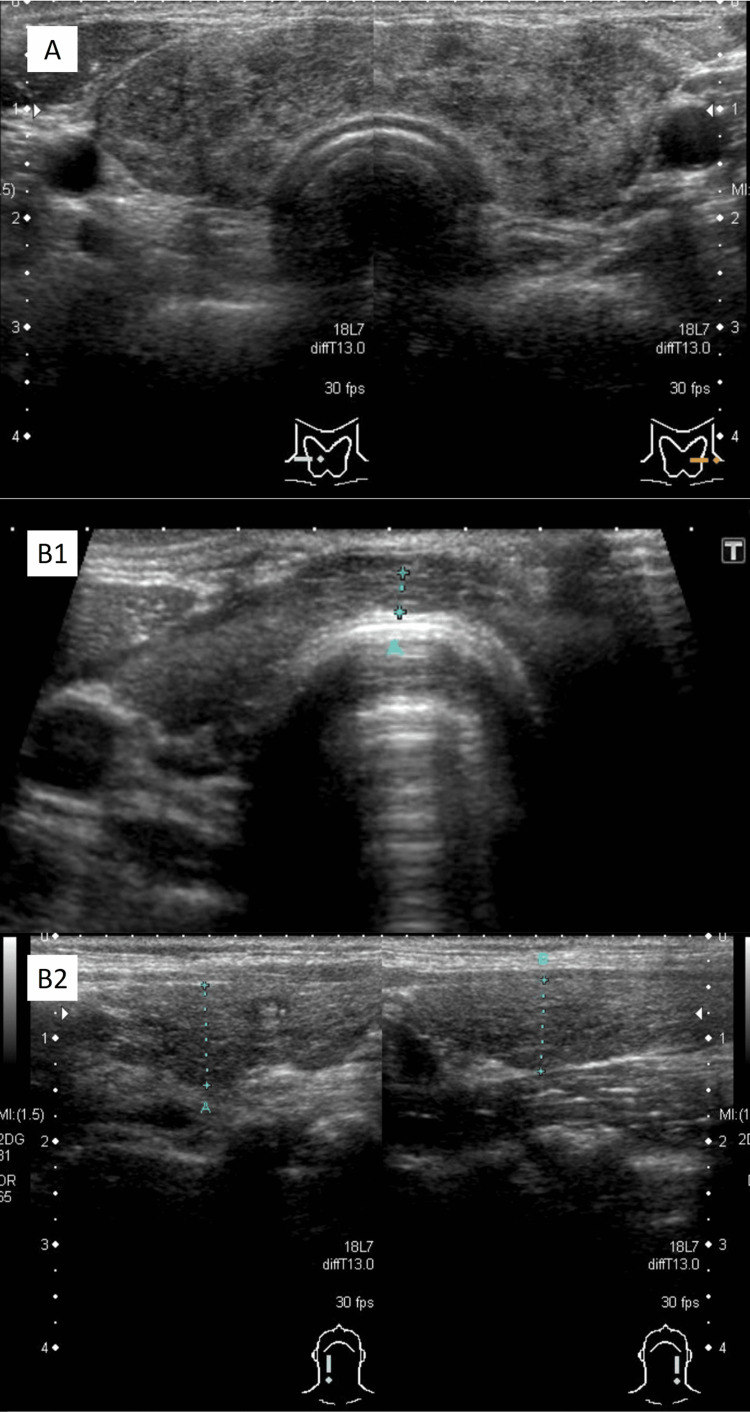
Thyroid ultrasound findings of Patient one. A: Day +103 (at onset), right lobe 19 mm, isthmus 7 mm, left lobe 19 mm B1, B2: Day +307 (at follow up), right lobe 10mm, isthmus 2 mm, left lobe 9 mm
